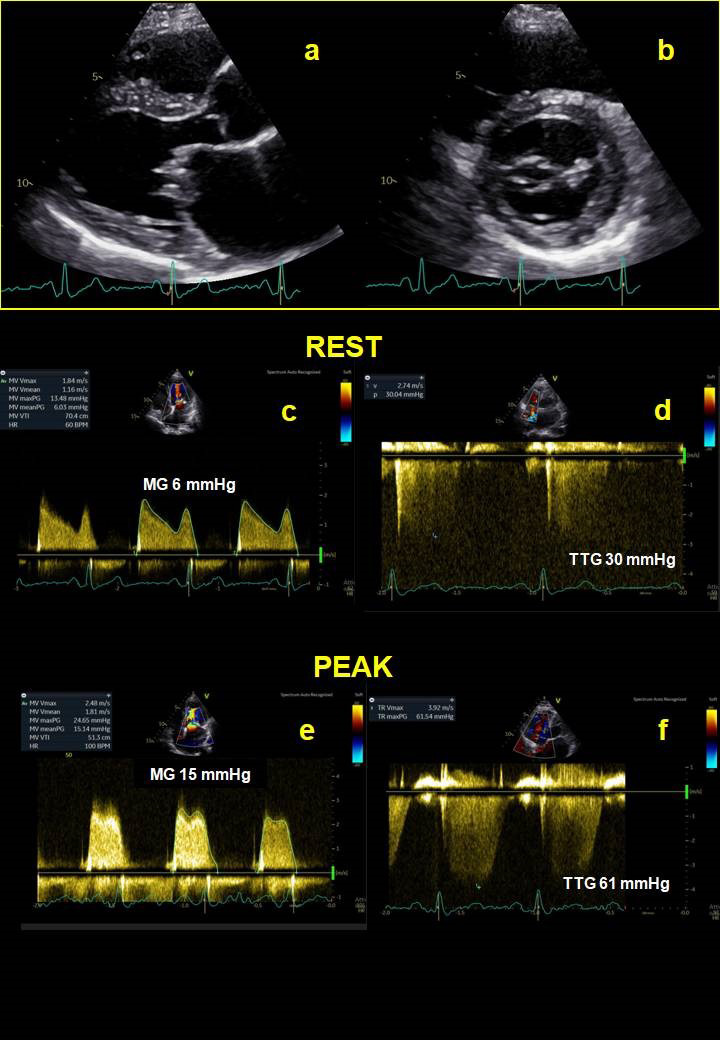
Mitral stenosis. Physical stress echocardiography of a 76-year
